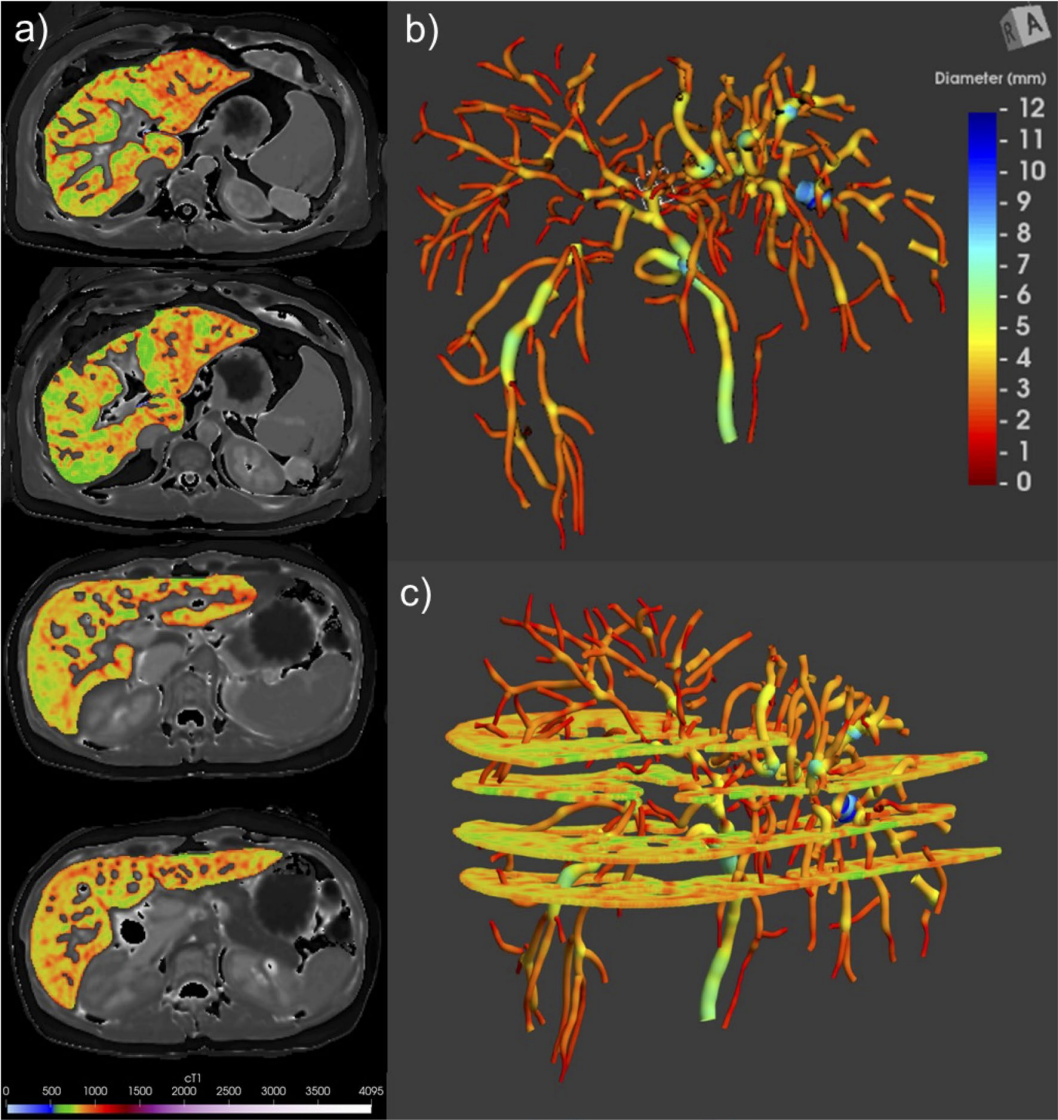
Co-registration of two quantitative MRI techniques for Pd-cT1 quantification in large-duct PSC. (a) Four segmented liver axial cT1 maps from Liver*MultiScan* analysis acquired at different levels with prominent blood vessels excluded and liver parenchyma colour-coded to liver cT1 values. (b) 3D biliary tree model derived from *MRCP+* analysis, colour-coded according to duct diameter size and orientation in geometric space depicted in the top right corner. (c) Co-registration of both images using MRI acquisition coordinates for the purpose of Pd-cT1 quantification
